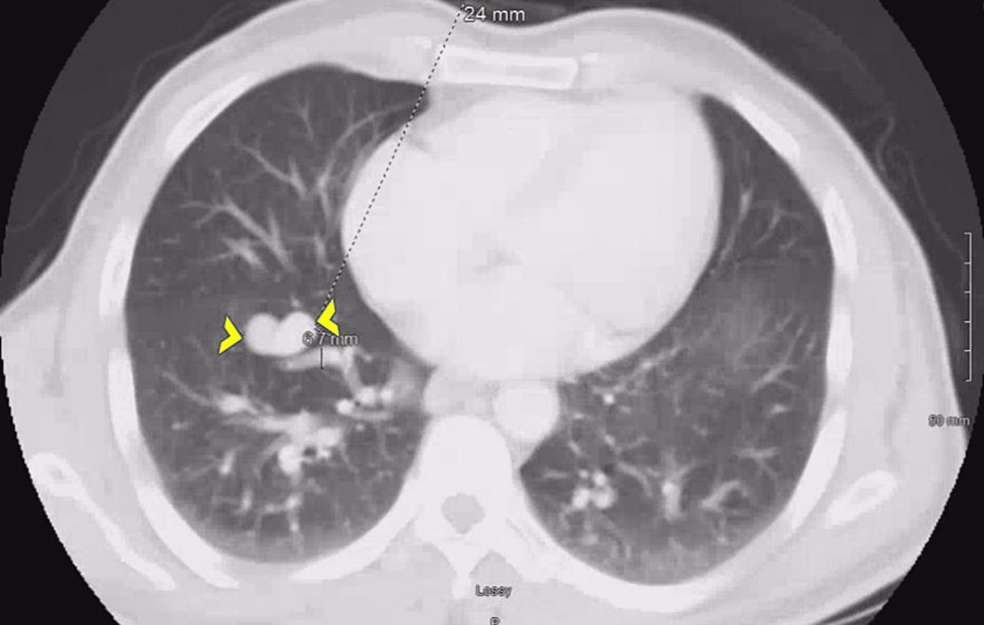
Computed tomography scan showing smooth lobular enhancing structure representing pulmonary arteriovenous malformation in the right lower lobe
